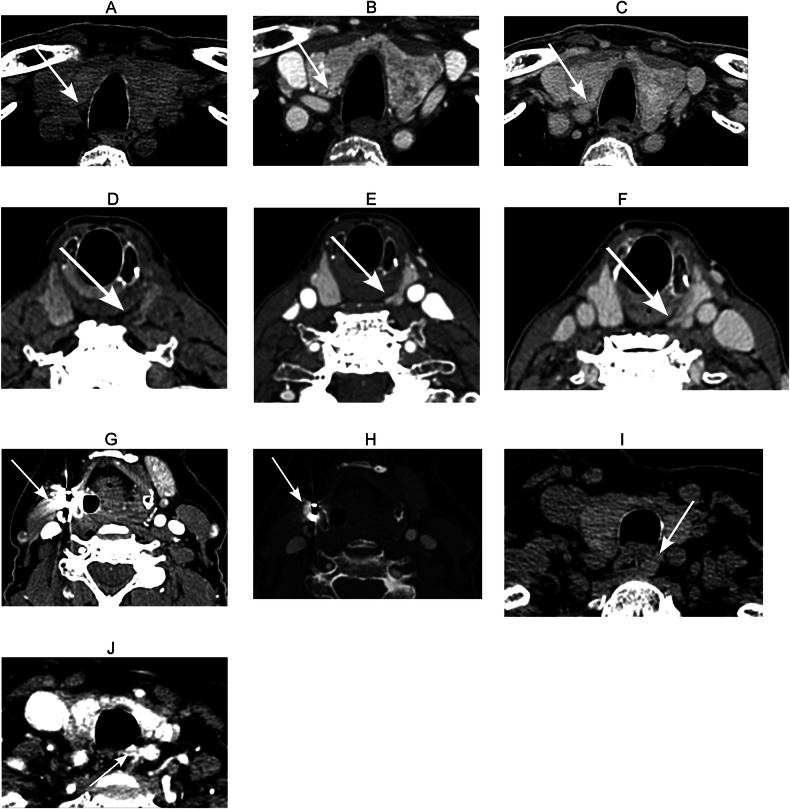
Examples of initially missed parathyroid adenomas on 4DCT in four patients. A–C Non-contrast (A), arterial phase (B) and delayed phase (C) images showing a parathyroid adenoma closely adherent to the right thyroid lobe, initially misinterpreted as thyroid tissue. The lesion appears slightly hypodense relative to the thyroid parenchyma on non-contrast CT and isoattenuating relative to the thyroid on the arterial phase and delayed phase (type C enhancement pattern). D–F Non-contrast (D) arterial phase (E) and delayed phase (F) images showing another parathyroid adenoma adherent to the left thyroid lobe, also initially misinterpreted as thyroid tissue; the lesion is slightly hypodense on non-contrast CT and isoattenuating on the arterial phase and delayed phase relative to the thyroid (type C enhancement pattern). G Arterial phase image of a parathyroid adenoma located anterior to the common carotid artery, not visualized due to metal artifact following prior thyroidectomy. H Same image with adjusted window settings, enabling retrospective visualization of the parathyroid adenoma. I, J Non-contrast (I) and arterial phase (J) images of a paraesophageal parathyroid adenoma, not detected because of motion artifact on the arterial phase
